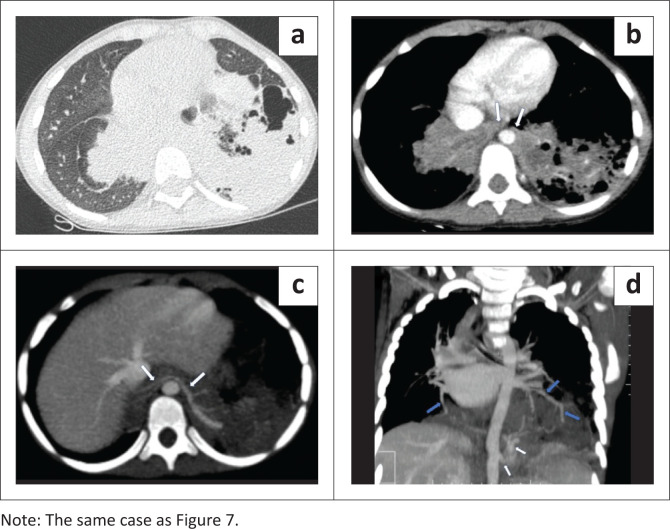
(a and b) Axial CT images (a, lung window and b, mediastinal window) confirm the presence of lung sequestration within the basal segments of both lower lobes, which are fused together in the midline anterior to the aorta (arrows). A partial accessory fissure is seen in the right lower lobe and consolidation with cavitation in the left lower lobe. (c) Axial maximum intensity projection (MIP) image shows an aberrant systemic arterial branch traversing through the bridging isthmus from left to right (arrows) and supplying the sequestered lung segments bilaterally. (d) Coronal MIP image demonstrates the origin of the systemic arterial branch (white arrows) from the abdominal aorta, as well as the area of consolidation in the left lower lobe. Bilateral inferior pulmonary veins (blue arrows) are draining the respective sequestered areas in each lung.
